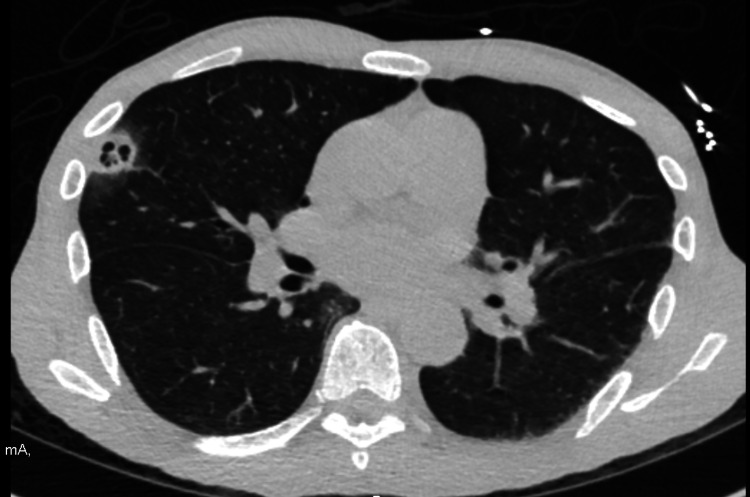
CT chest shows peripheral cavitary opacities in the right upper lobe
